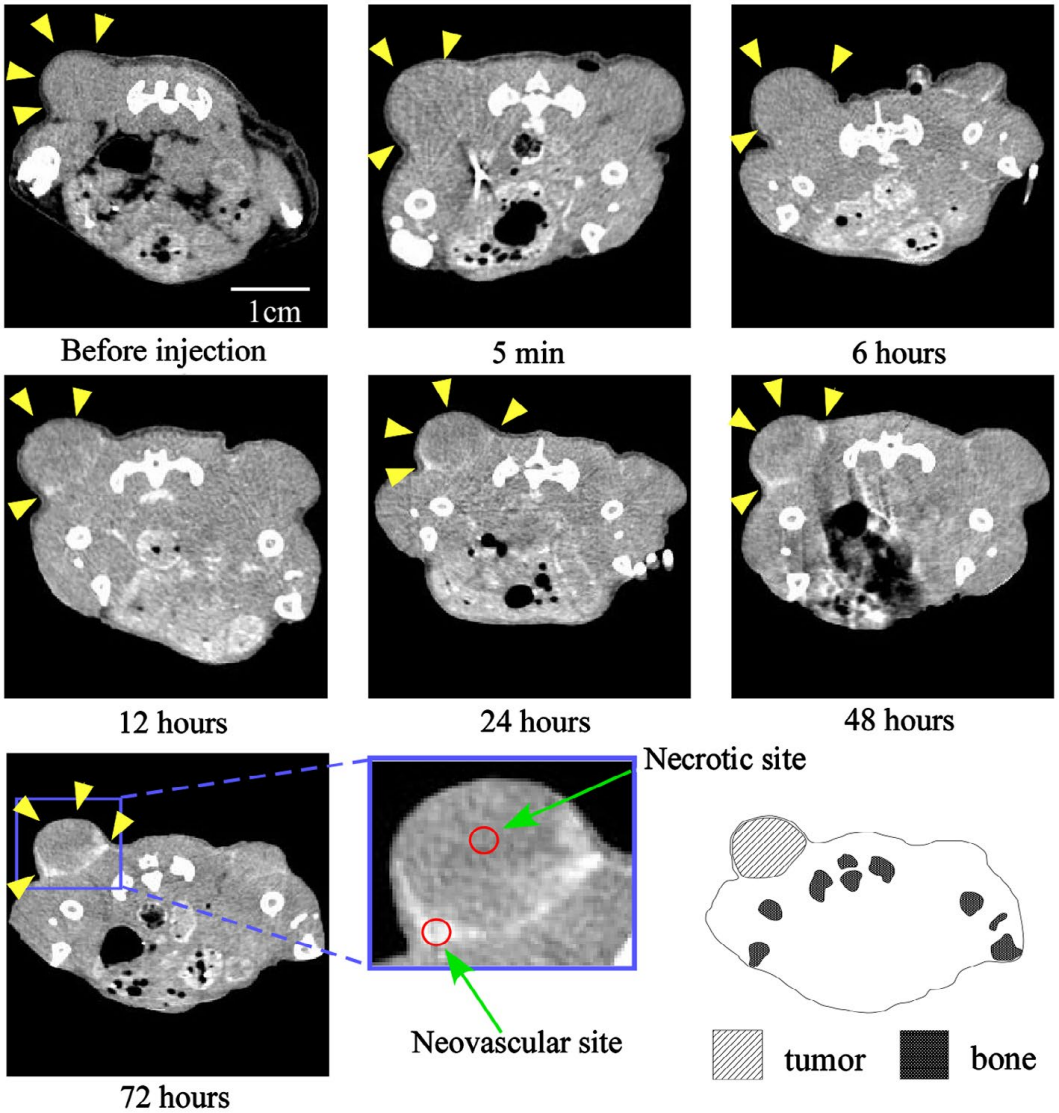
Contrast effect within a tumor after the injection of 30-nm Au-PEG. The tumor signal was greatly enhanced, and the tumor border was clearly imaged. Yellow arrowheads demonstrate the tumor region. The CT values of the regions of interest (ROIs), which are shown in red circles, were measured. The mean CT values of the necrotic site (tumor center) and neovascular site (tumor border) were 63 ± 12 HU and 235 ± 22. HU, respectively.
